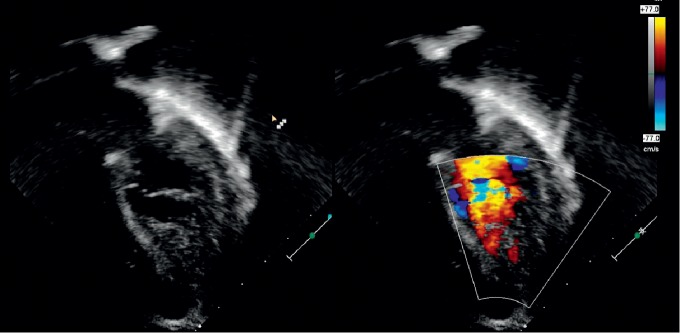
Four-chamber ECHO with color Doppler showing a spongy looking myocardium with dilated sinusoids suggestive of left ventricular noncompaction.
